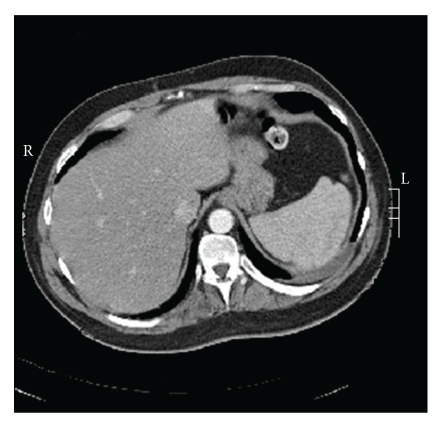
Post-op contrast CT abdomen showing normal abdominal aorta at its origin.
